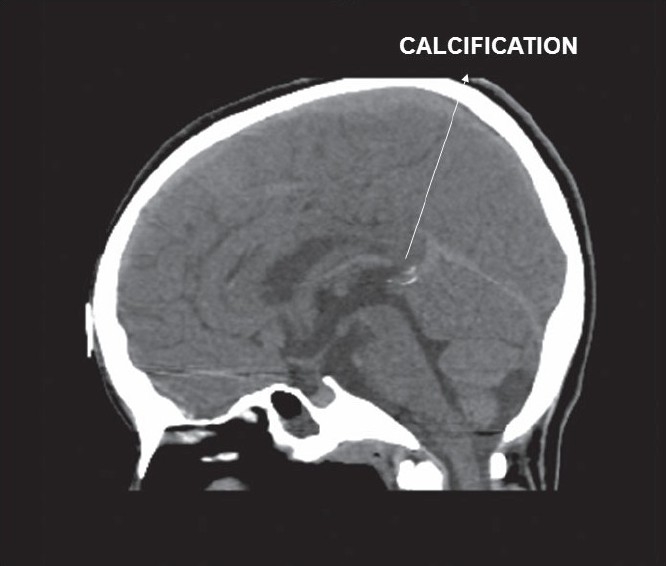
Sagittal CT image of the brain showing calcification in the globus pallidus
